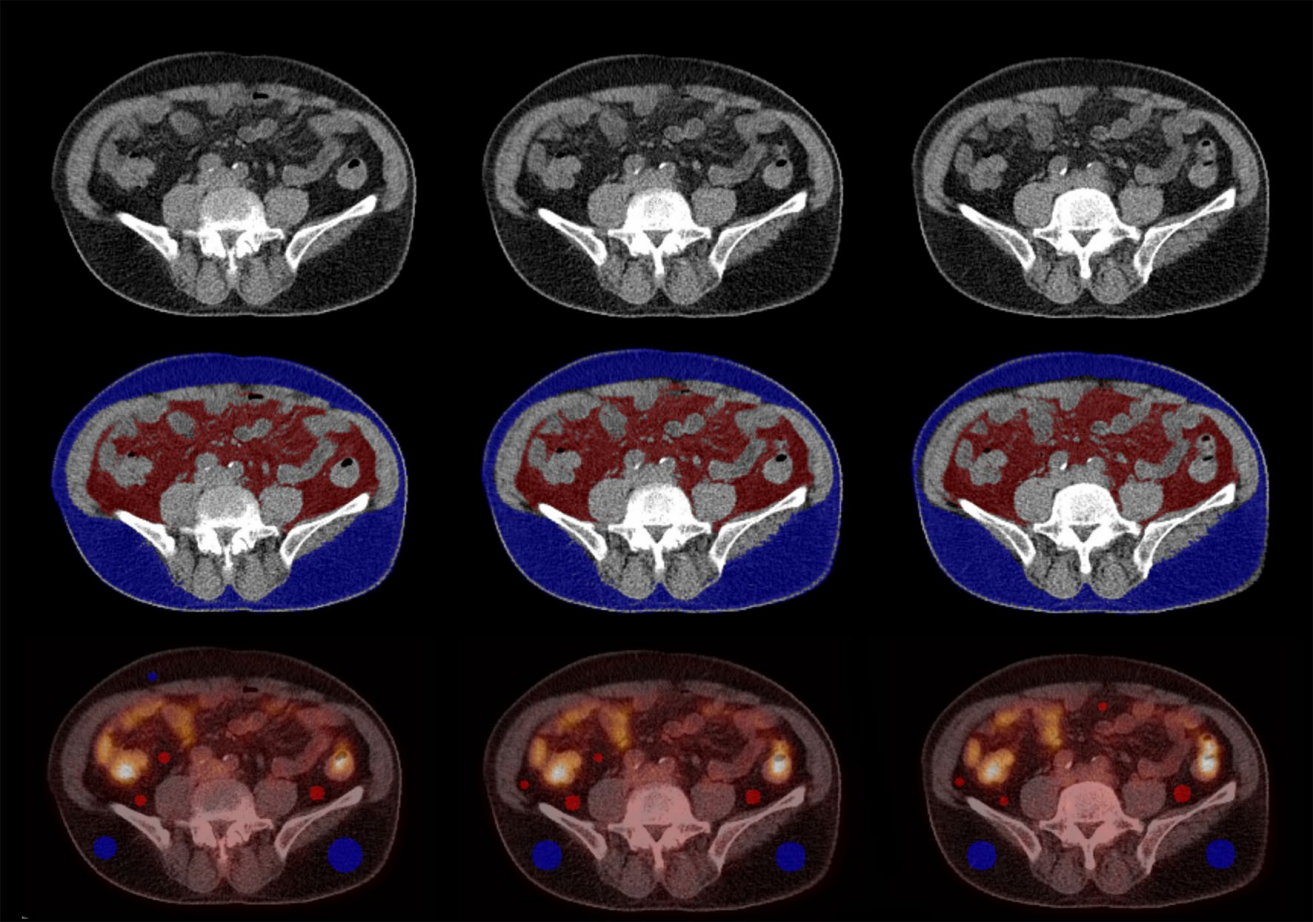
Example of measurement of the volume and standardized uptake value of visceral and subcutaneous tissue from PET/CT images. On the three consecutive trans-axial CT images at the level of L4/5 (top row), the volume of VAT (red) and SAT (blue) were automatically delineated using CT-attenuation range between − 190 and − 30 HU (middle row). On fused PET/CT images, circular ROIs were manually placed on the VAT area while avoiding the spillover from adjacent structures (bottom row). Similarly, circular ROIs were placed on the SAT area (bottom row).
